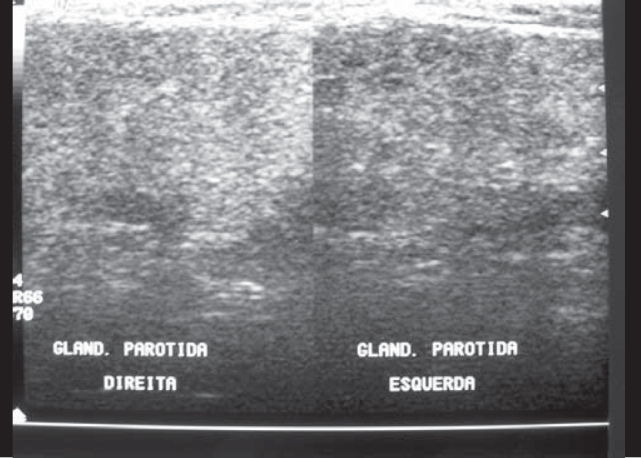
FDO Ultrasound (case 1). Initial examination. Left gland presented heterogeneous texture and the right gland was unaltered.
